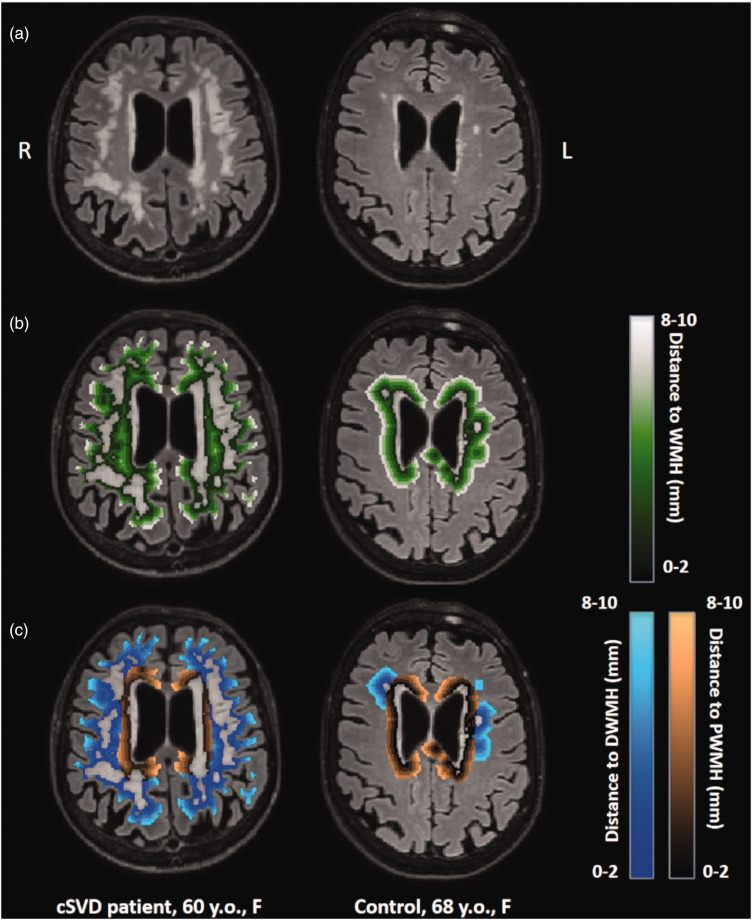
(a) T2-weighted FLAIR scans with white matter hyperintensities (WMH) for a 60-year-old female cSVD patient (left) and for a 68-year-old female control (right). (b) Visualization of 2-mm-thick shells around all WMH and (c) visualization of 2-mm-thick shells around the periventricular WMH (PWMH) and the deep WMH (DWMH).
